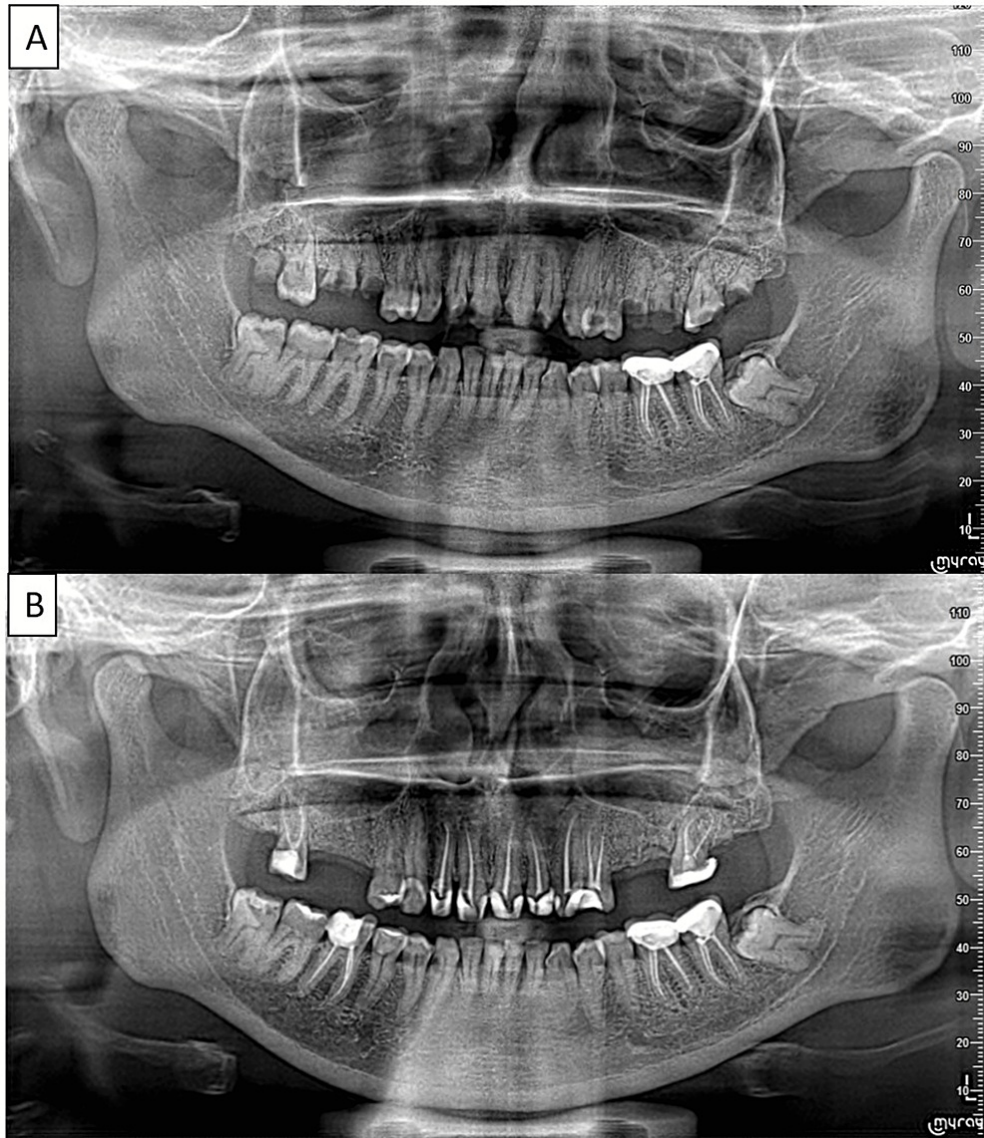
(A) Preoperative OPG; (B) post-RCT OPG. OPG, orthopantomogram; RCT, root canal treatment
